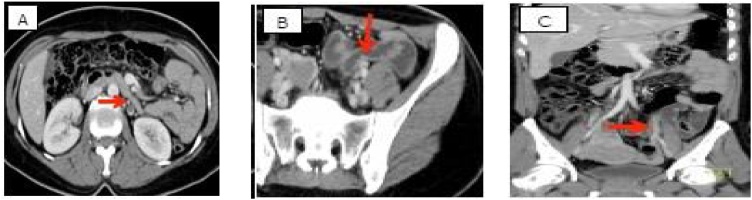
(A,B&C): CT scan with contrast of the Abdomen showing left ovarian vein thrombosis.
